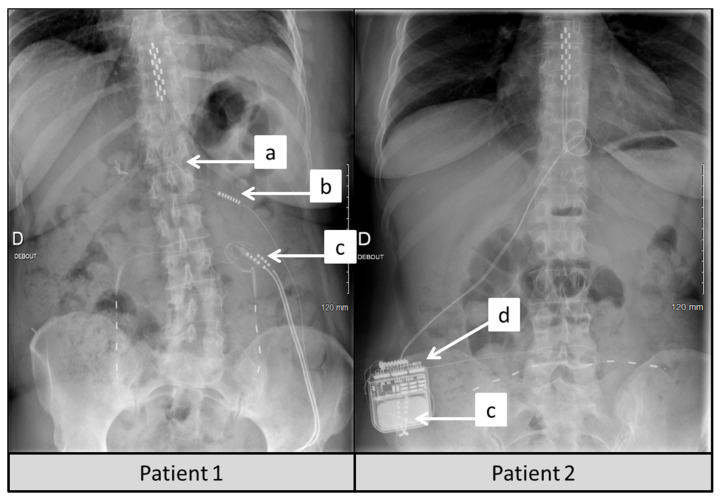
Post-operative X-ray showing technical considerations of SCS + PNfS combination. (a) For this first patient, the first channel of the surgical lead wire was disconnected at the lead-extension junction, after selecting the “best” channel to maintain adequate leg pain coverage by reprograming, between the two. (b) The second channel, selected as “the best channel” remained connected to the IPG via port *n*2. (c) A “Y” extension was implanted to connect the PNfS leads, which were implanted subcutaneously (Quad Plus 4-contact leads, Medtronic) to the 8-contact port *n*1 of the IPG. (d) Similarly to Patient 1, the IPG (Restore Advanced, Medtronic) delivers electricity through one channel dedicated to SCS and one other channel dedicated to PNfS for Patient 2.
